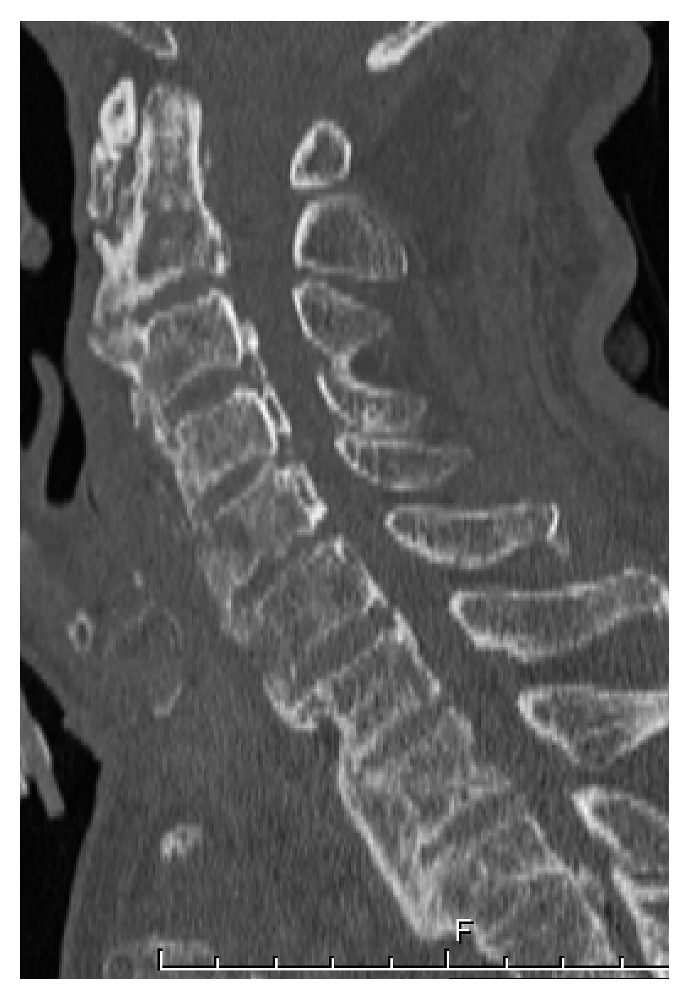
Sagittal CT image after removal of OALL.
